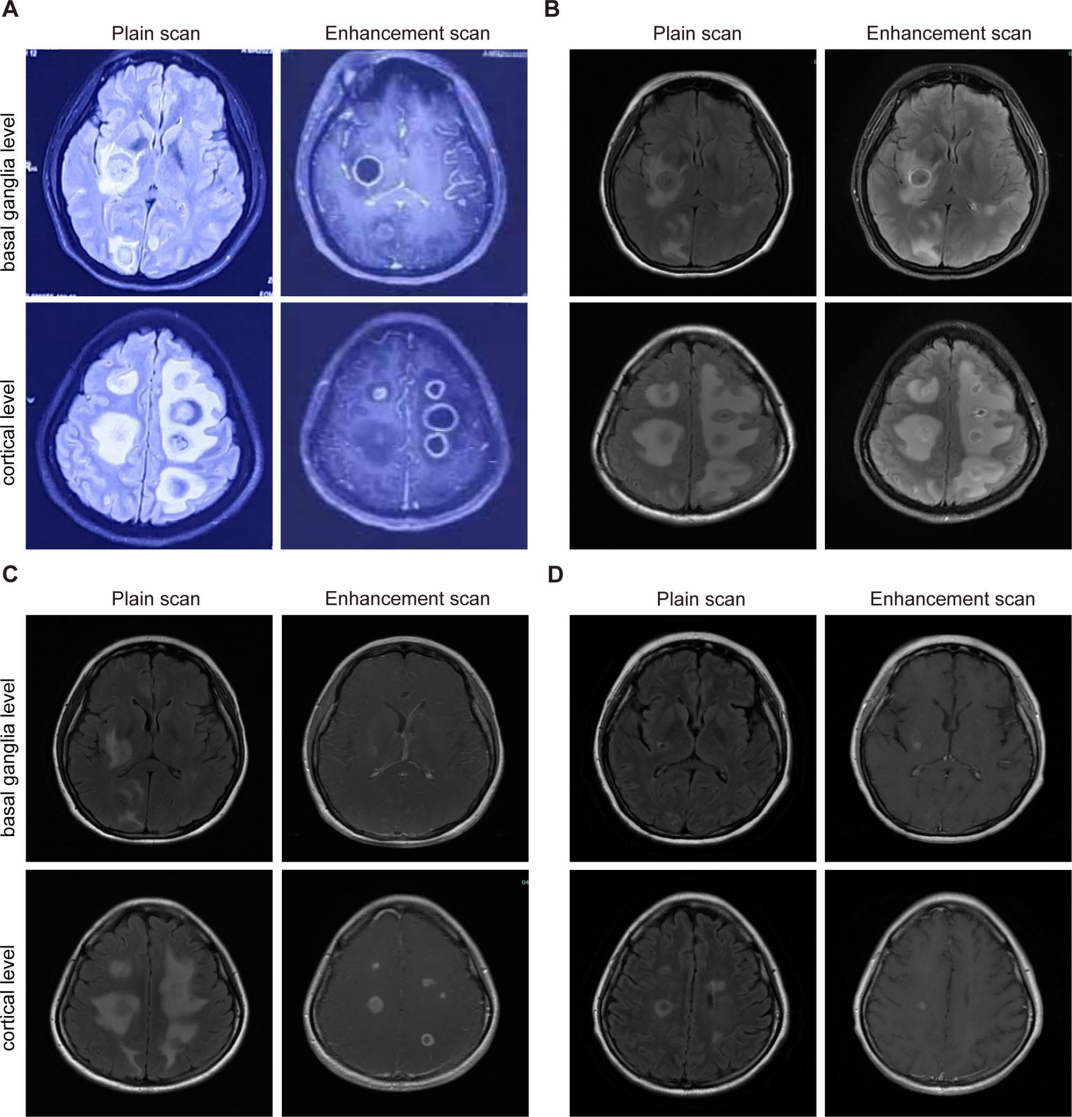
Head MRI at different stages. (A) Head MRI (13 days after the accident) showed multiple ring reinforcement nodules and surrounding edema in the brain. (B) On March 7, 2023(1 week of antifungal treatment), the head MRI showed that the brain abscess lesions were smaller than before, and the edema around the lesions was more serious. (C) On April 6, 2023(5 weeks of antifungal treatment), a head MRI showed that the abscess lesions were smaller and the edema was lighter than on March 7, 2023. (D) On October 16, 2023(more than 7 months of antifungal treatment), the brain abscess lesions still exist
